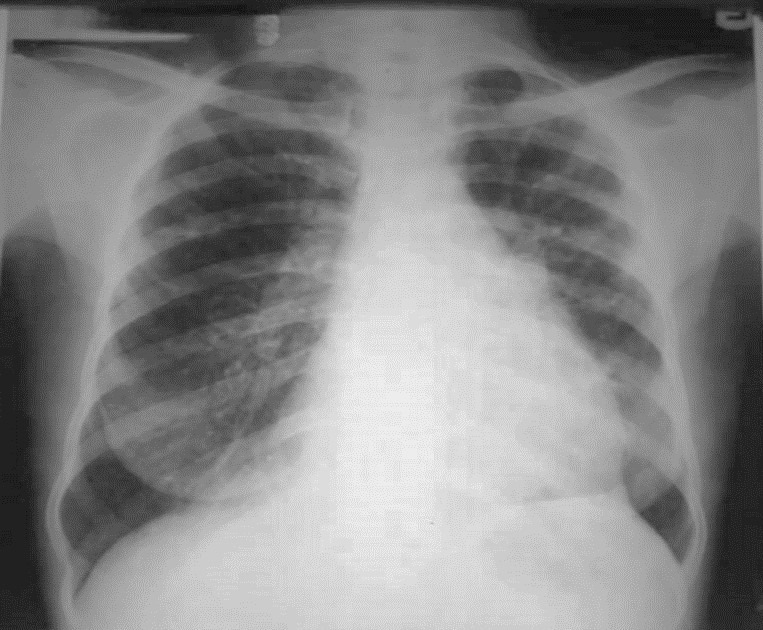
Chest X-ray showing moderate cardiomegaly with mild vascular engorgement with perivascular cuffing
